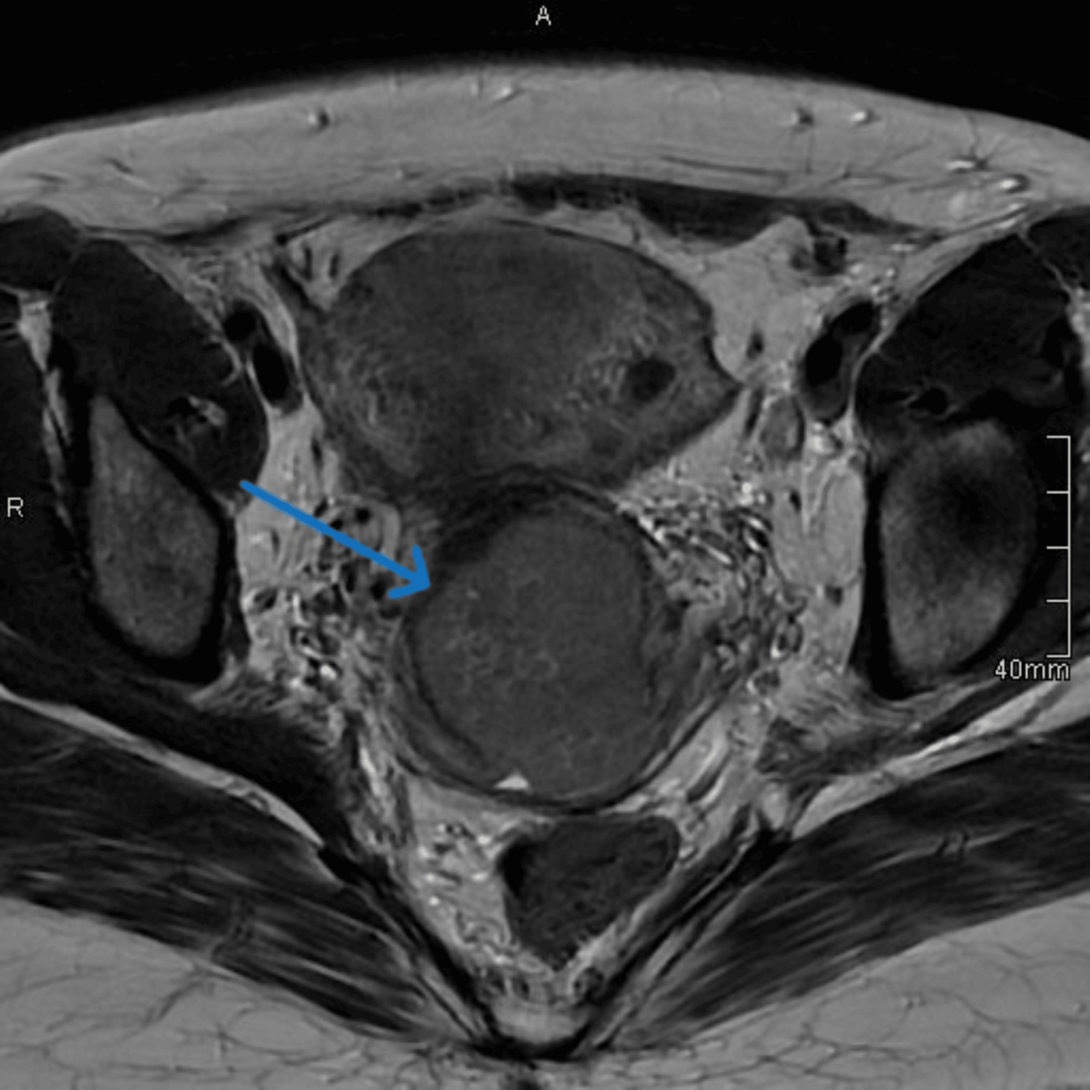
Axial T2 MRI of the pelvis demonstrates a large cervical mass with parametrial invasion.
